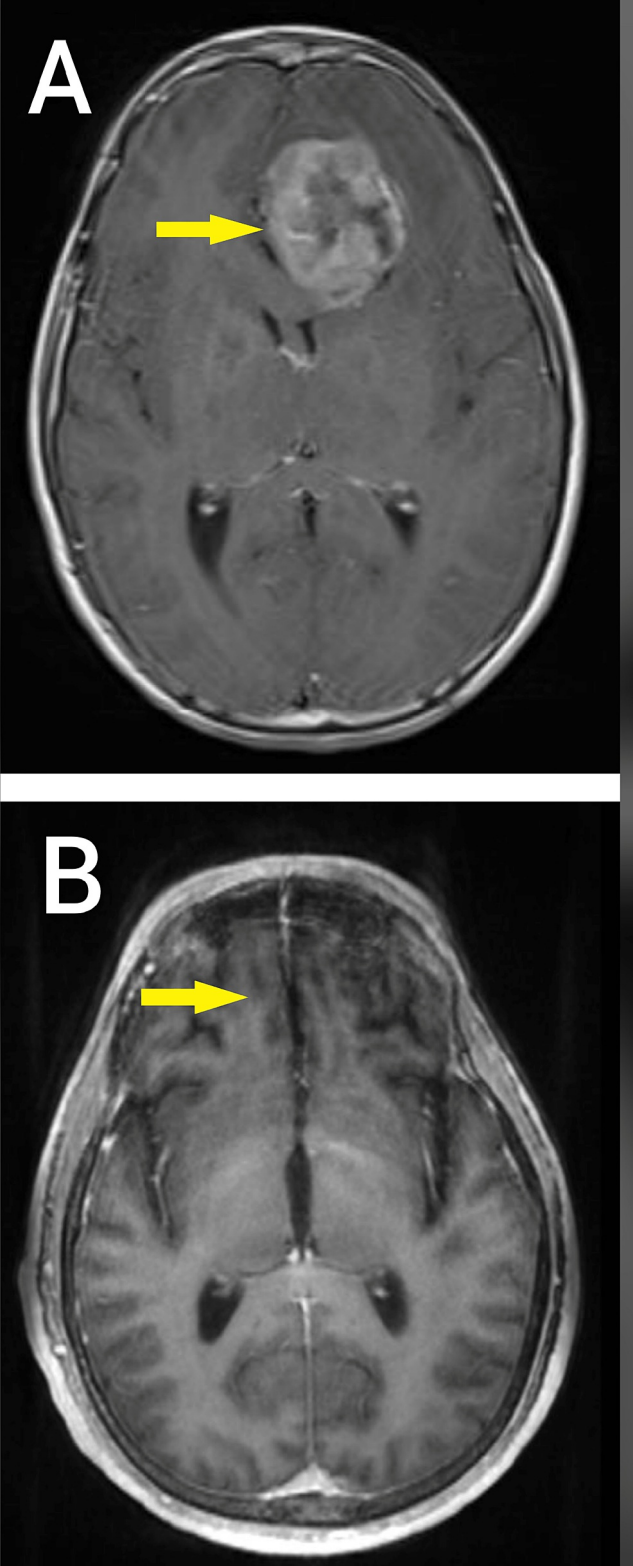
T1-weighted MRI images: (A) Pre-operative MRI dated on 6.7.2008 revealed evidence of a large left frontal intra-axial mass lesion, shown by the arrow arising medially and extending towards the genu of the corpus callosum, associated with irregular enhancement. There is a mass effect with displacement of the midline to the right side and effacement of the anterior horn of the left lateral ventricle. (B) MRI dated on 11.10.2021, revealed evidence of post-surgical changes in the form of mild gliosis shown by the arrow; otherwise, no mass lesion is shown.
